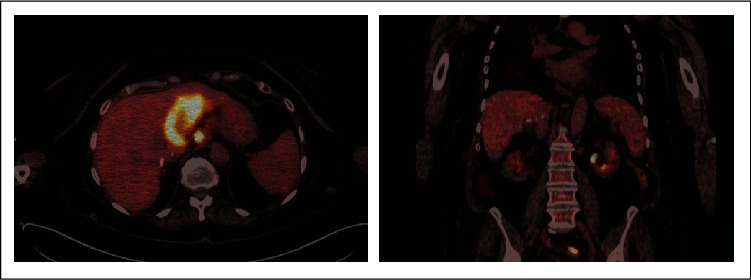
Posttreatment. PET-CT: significant decrease in the metabolism in the spleen, after 4 doses of tocilizumab.
